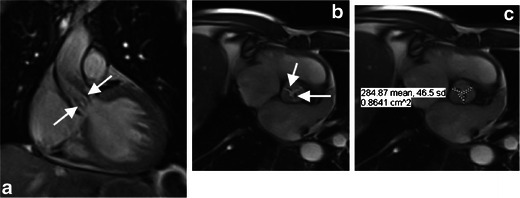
A 66-year-old male with aortic stenosis. a Steady-state free precession MR image at peak systole in coronal plane shows hypointense jets in the aortic root (*white arrows*), which result from dephasing of turbulently flowing blood, consistent with haemodynamically significant aortic valve stenosis. b Steady-state free precession MR image at peak systole in short axis plane of aortic valve shows a tricuspid aortic valve with valve leaflet thickening because of sclerosis and/or calcifications (*white arrows*). c Planimetry in the short axis plane of the aortic valve at peak shows maximal valve opening of 0.86 cm², consistent with severe aortic valve stenosis. MR imaging is particularly useful for TAVR candidates with renal failure since necessary measurements can be obtained without administration of gadolinium
